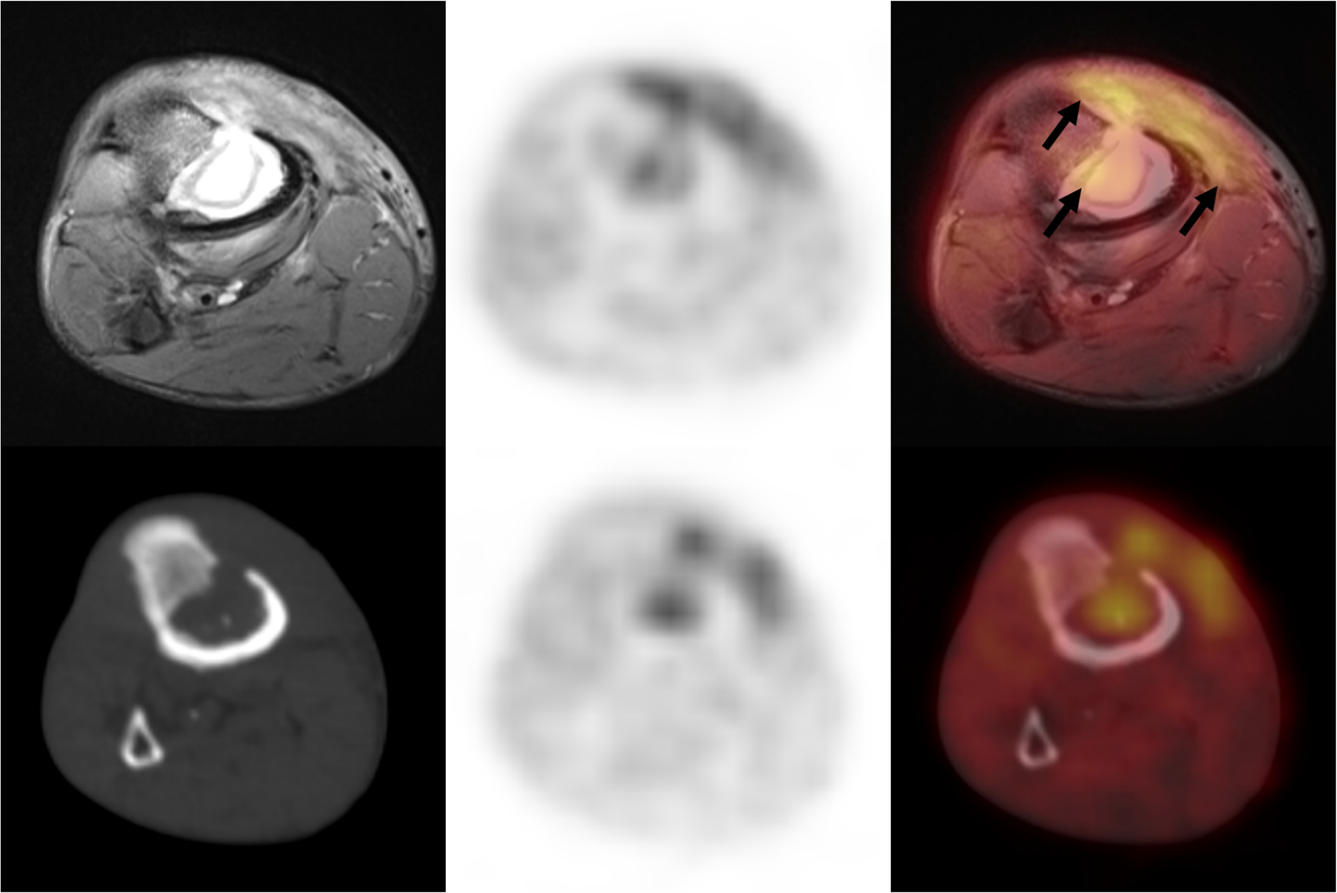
Reconstructed axial images of the affected region (left tibia) from patient 1. Top row, left to right: T2-weighted MRI, PET component of PET/MRI, and hybrid PET/MRI overlay. Bottom, from left to right: CT, PET component of PET/CT, and hybrid PET/CT overlay. Black arrows denote on hybrid PET/MRI, and the area of high FDG uptake can clearly be demarcated by soft tissue features that are depicted by MRI
